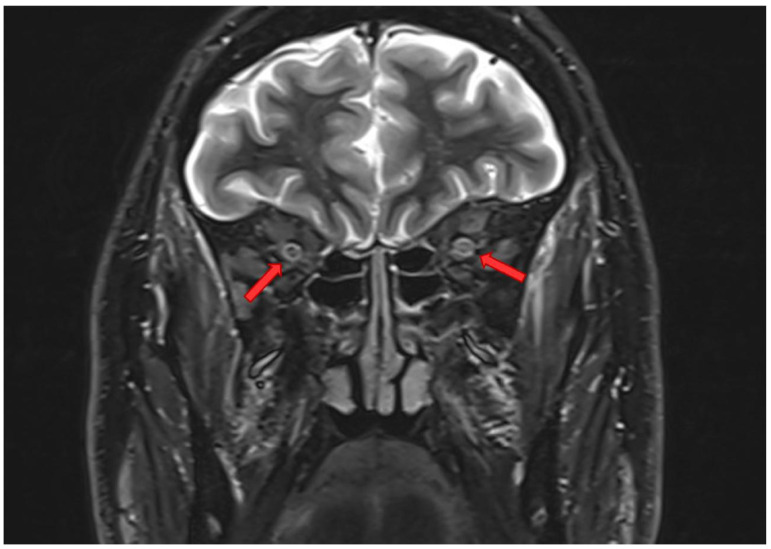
FLAIR MRI showing bilateral hyperintense signal of the optic nerves, consistent with acute bilateral optic neuritis. Arrows indicate the Hyperintense and swollen intracanicular segments of both optic nerves.
